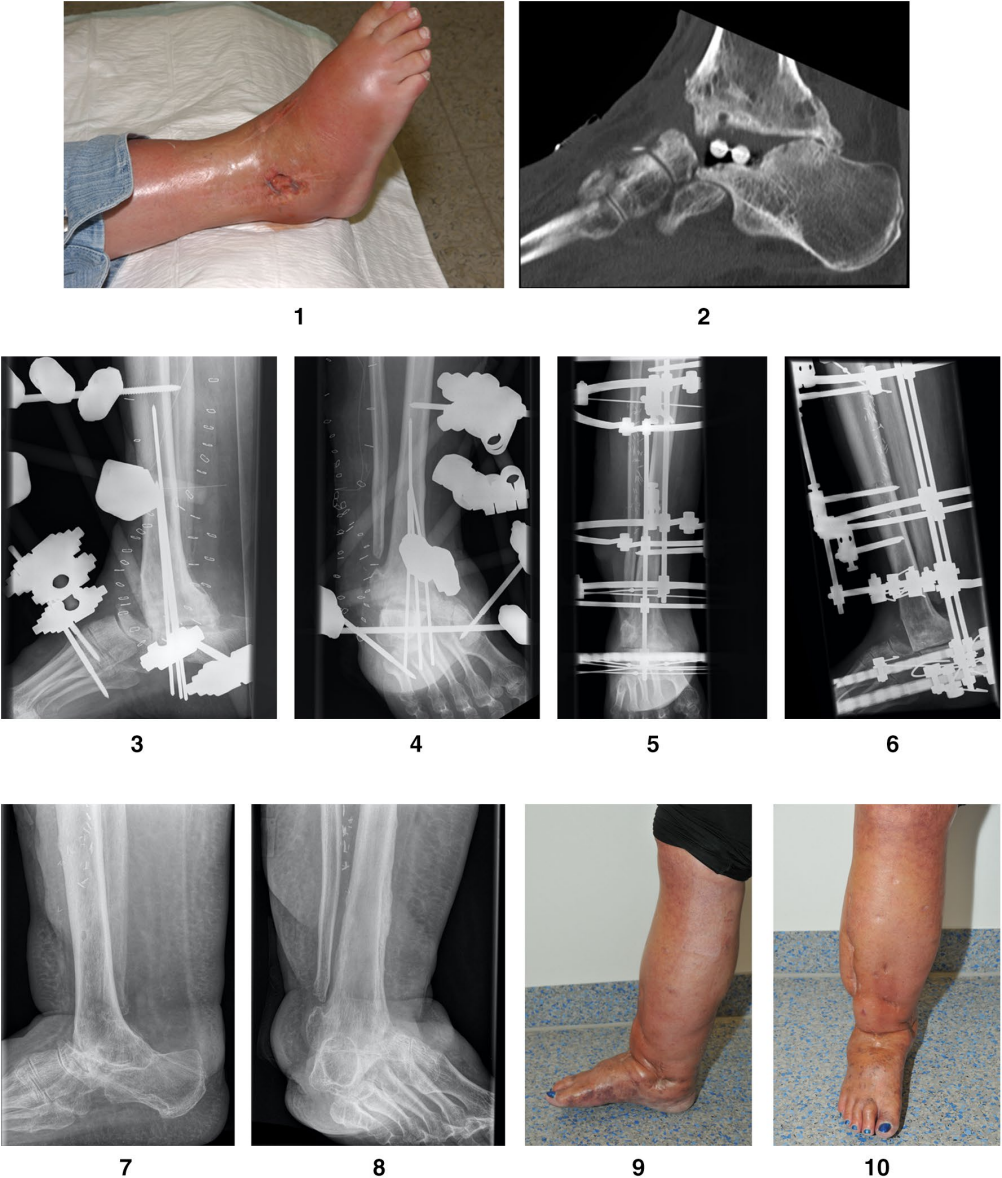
Figs. 1, 2: Patient 1—a 49-year-old woman presented with florid infection and destruction of the subtalar joint and talus and preexisting arthrodesis of the ankle after an initial fracture and multiple operations. When the patient was referred to our hospital, antibiotic chains were still present in the subtalar joint. Figs. 3, 4: Patient 1—x-ray image after talus resection, extensive debridement and placement of an AO fixator. The talus was completely destroyed due to infection, which is why it was completely removed. After initial VAC therapy, a plastic flap covering with the anterior lateral thigh (ALT) flap was necessary. Figs. 5, 6: Patient 1—after the soft tissue was successfully covered, the Ilizarov fixator was installed. Figs. 7, 8: Patient 1—after the fixator had been worn for 33 weeks, it was removed and consolidation was observed. Figs. 9, 10: Patient 1—clinical picture at the last follow-up examination after 121 months. The woman was able to walk, and the AOFAS score was 62 of 86 possible points
